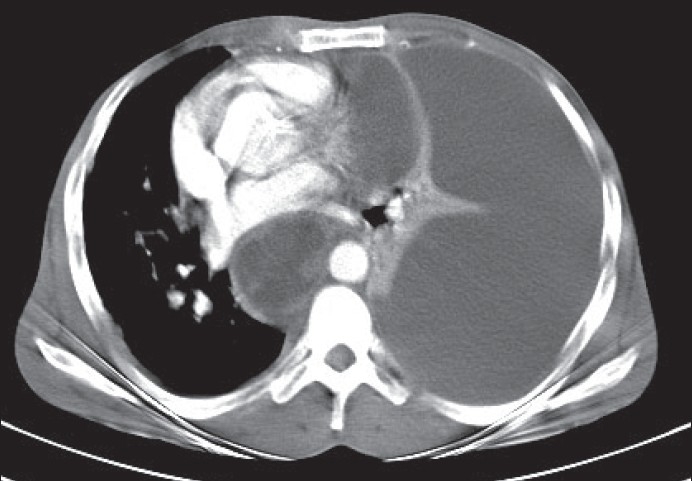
CT scan thorax showing massive left-sided pleural effusion with compressive collapse of left lung and a posterior mediastinal cyst
